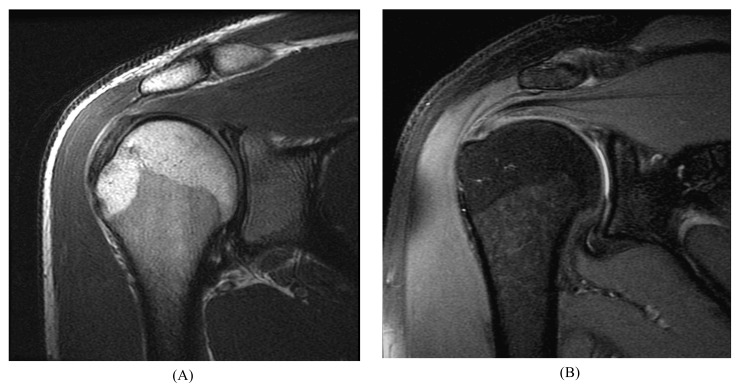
SLAP tear. Laterally curved linear signal located posterior to the biceps anchor in (A) a 22 year-old collegiate baseball pitcher, on a coronal intermediate-weighted image and (B) a 27 year-old overhead athlete, on a coronal fat-suppressed T2-weighted image.
